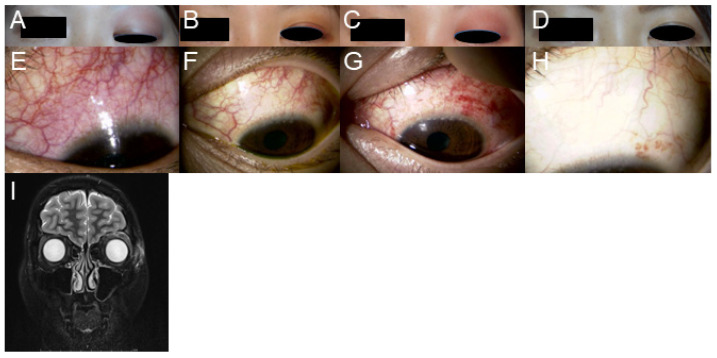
(A). Eyelid findings at the initial examination. Upper eyelid swelling is observed. (B). Eyelid findings 3 days after the initial examination. Upper eyelid swelling improved with antibacterial and steroid eye drops. (C). Eyelid findings 1 month after the initial examination. Upper eyelid swelling worsened again. (D). Eyelid photograph 4 months after systematic steroid treatment. The eyelid swelling resolved. (E). Anterior segment of the eye at the initial examination. Episcleritis is observed in the upper eye. (F). Anterior segment of the eye 3 days after the initial examination. Hyperemia in the upper eye improved with antibacterial and steroid eye drops. (G). Anterior segment of the eye 1 month after the initial examination. Episcleritis exacerbated again. (H). Anterior segment of the eye 4 months after steroid treatment. No hyperemia was observed, and episcleritis resolved. (I). Orbital magnetic resonance image (short TI inversion recovery) at the initial examination. Edematous signal changes are observed in the left eyelid and orbicular muscle. Periorbital cellulitis was suspected.
